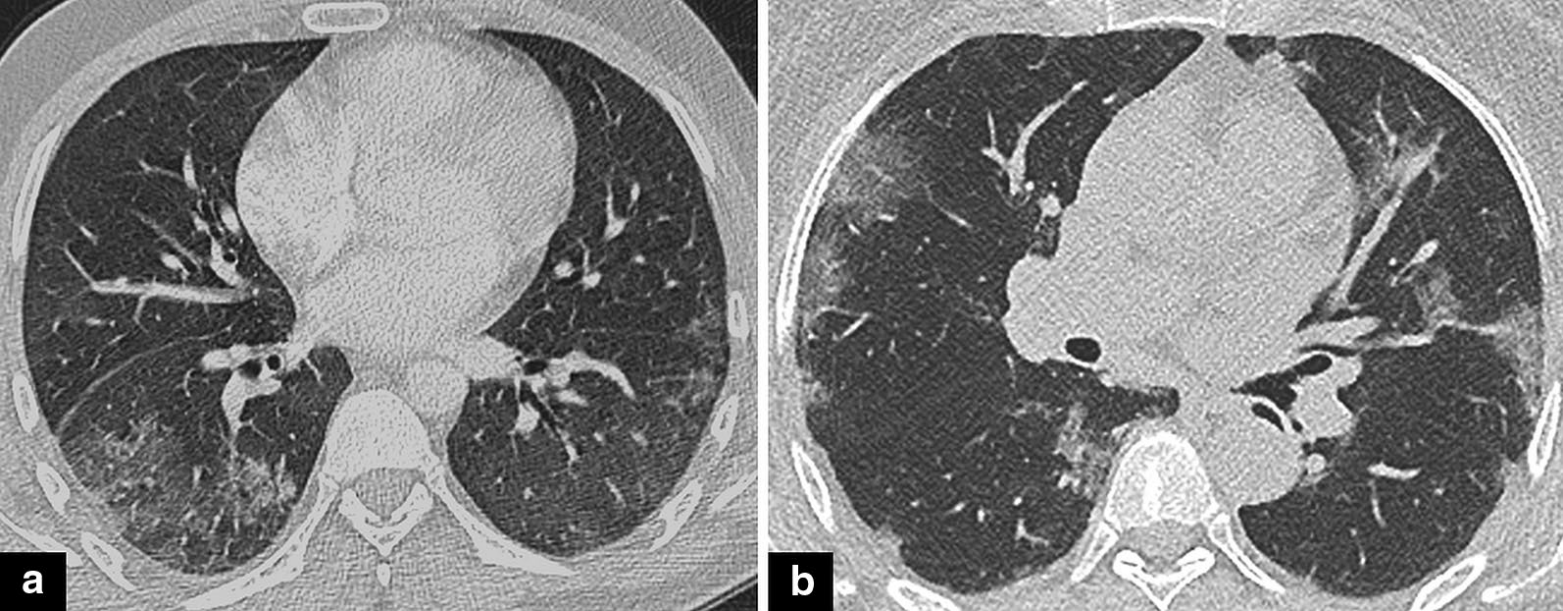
Drug toxicity and COVID-19 pneumonia. a Bilateral peripheral GGOs are observed in the axial CT image of a patient receiving bleomycin chemotherapy for testicular malignancy. Following the discontinuation of the drug, opacities were completely resolved. b In a patient diagnosed with COVID-19 pneumonia, bilateral multiple GGOs resembling (a) are seen
